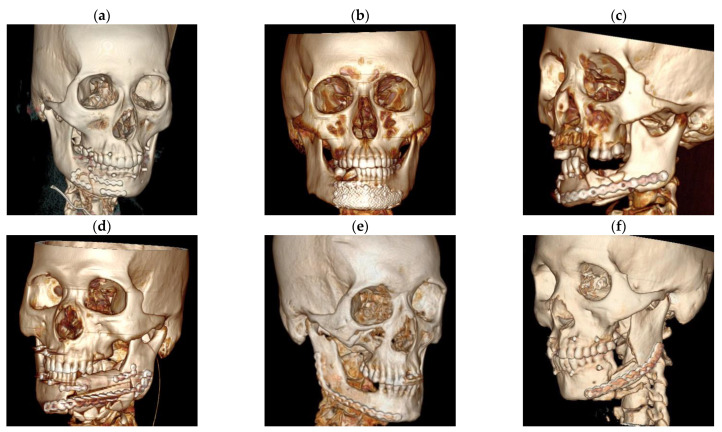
Postoperative 3DCT reconstruction of each fracture category. (a) Type II fracture managed with miniplates fixation following the Champy’s principle; (b) type III fracture, MMF, staged surgeries and titanium mesh fixation were performed; (c) type IV fracture managed with reconstruction plate systems fixation following the AO/ASIF principles; (d) reconstructed with fibular microvascular osteocutaneous free flap; (e) reconstructed with scapular microvascular osteocutaneous free flap; (f) reconstructed with iliac microvascular osteocutaneous free flap. 3DCT: three-dimensional computed tomography; MMF: maxillomandibular fixation; AO/ASIF: Arbeitsgemeinschaft fuer Osteosynthesefragen/Association for the Study of Internal Fixation.
